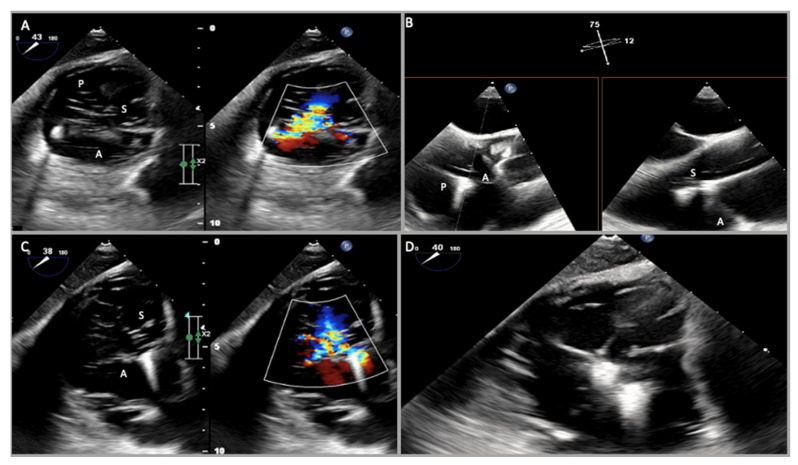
Intraprocedural monitoring by transesophageal echocardiography. (A) Transgastric short-axis view. Color Doppler is useful to guide positioning of the clip over the desired location relative to the regurgitant orifice. (B) Mid-esophageal commissural biplane view and (C) transgastric short-axis view: the position of the clip delivery system (CDS) with respect to the target lesion can be checked and corrected using 2D (single or biplane) and Color Doppler. (D) Transgastric short-axis view. The quality of leaflet grasp is verified by the limited leaflet mobility relative to the tips of the clip arms. A = anterior leaflet, S = septal leaflet, P = posterior leaflet.
